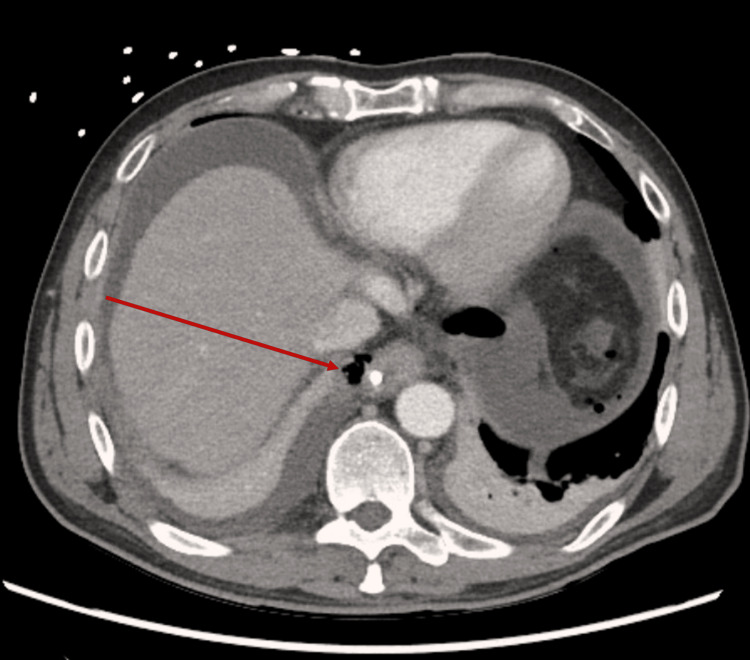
Axial computed tomography image demonstrating nasogastric tube perforating the distal esophagus with associated free air (red arrow).
